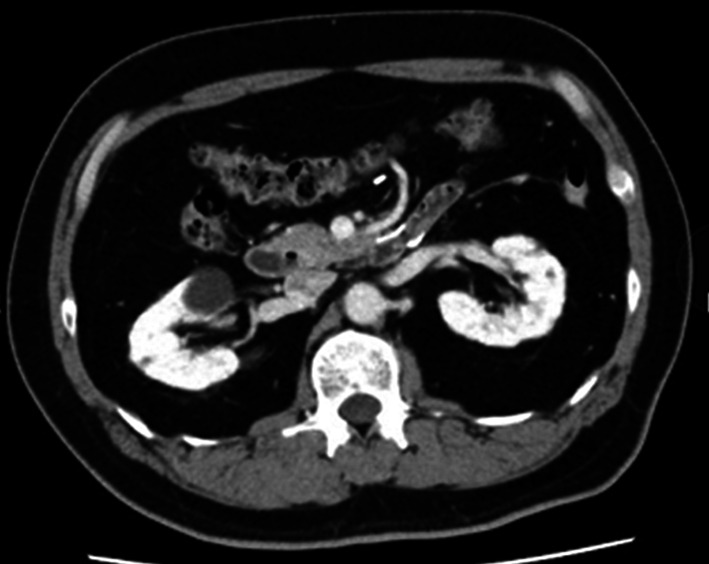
Contrast-enhanced computed tomography findings 2 years after surgery. Neither signs of recurrence nor stenosis has been observed
